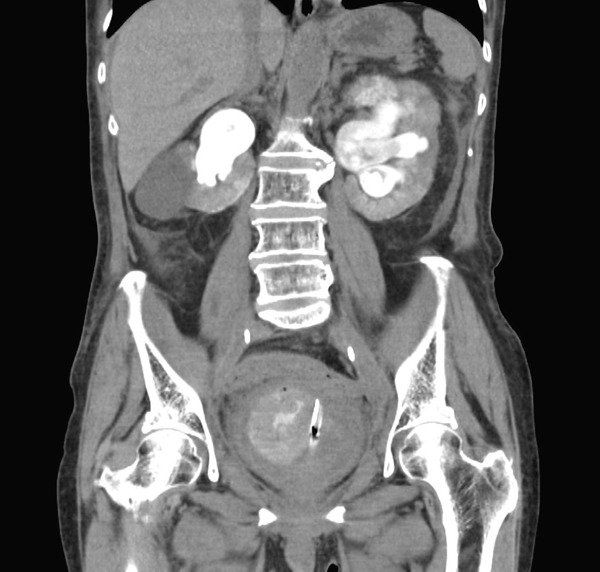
Contrast CT image after intermittent bleeding. A hematoma filling the left upper tract, with no evidence of arterial hemorrhage.
